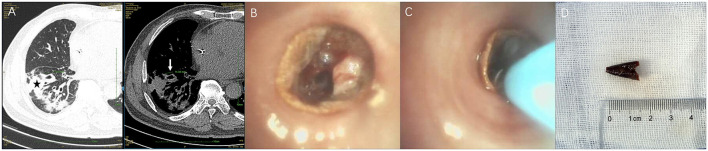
Radiolucent organic AFB, RLL. (A) CT: saccular bronchial dilation (white arrow, left) and adjacent consolidation (black asterisk, right). (B) Thin bronchoscopy: chili-pepper tip lodged in anterior RLL. (C) Cryoprobe inserted for extraction. (D) Retrieved 1.5 cm chili-pepper tip matching the dilated segment on CT. AFB, airway foreign body; CT, computed tomography; RLL, right lower lobe.
